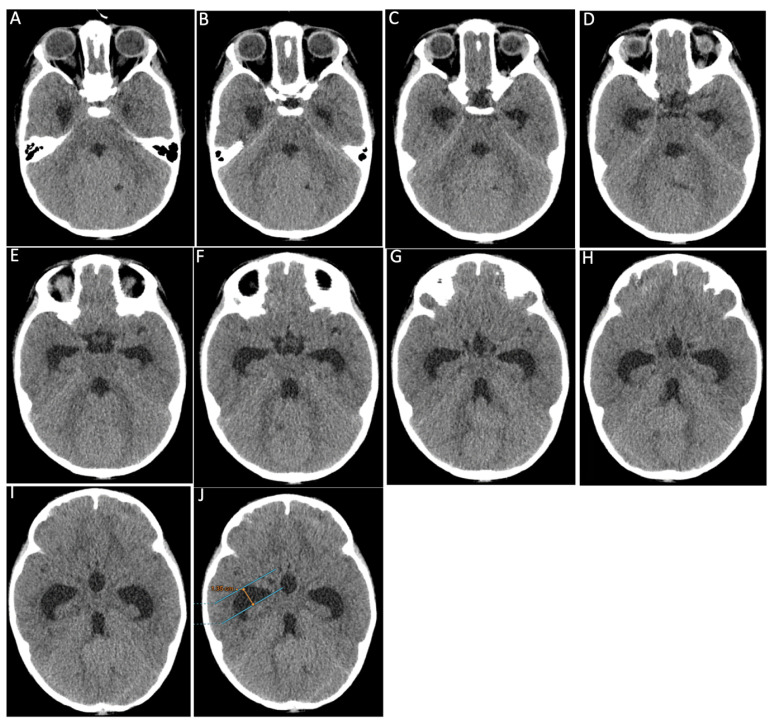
Ten contiguous 2-mm-thick CT axial slices
